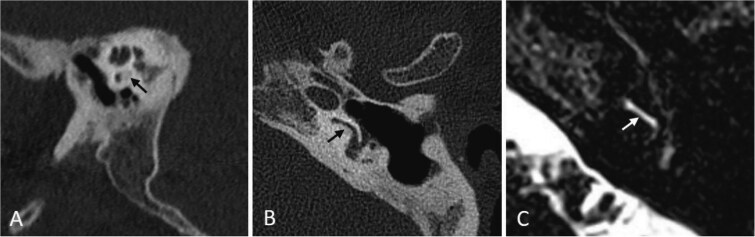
Ossified scala tympani in the basal turn of the left cochlea shown on sagittal (A) and axial (B) sections of high-resolution CT (black arrows). (C) On T2 sequences of MRI scan the ossification is causing missing hyperintensity under the scala vestibuli (white arrow).
